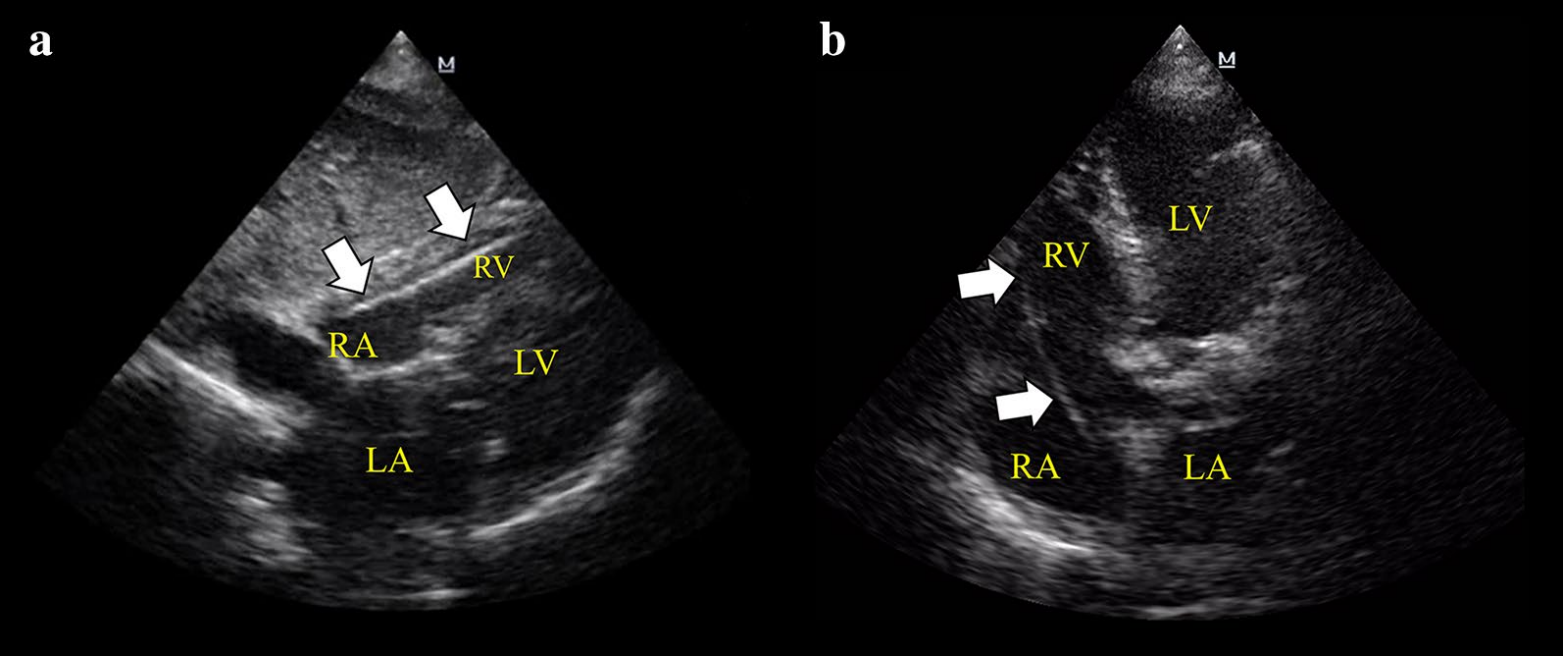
Subcostal 4-chamber view (a) and apical 4-chamber view (b) demonstrating the temporary pacing electrode catheter (EC, arrows) into the right ventricle (RV). *RV* right ventricle, *RA* right atrium, *LV* left ventricle, *LA* left atrium
